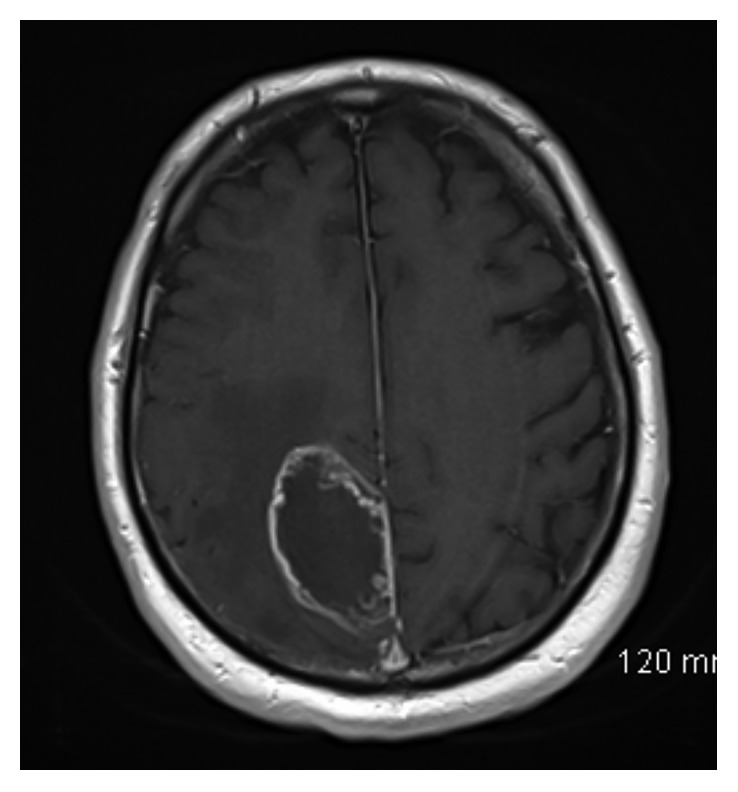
Axial T1-weighted image after administration of gadolinium demonstrating peripheral nodular enhancement of right parietal mass.
